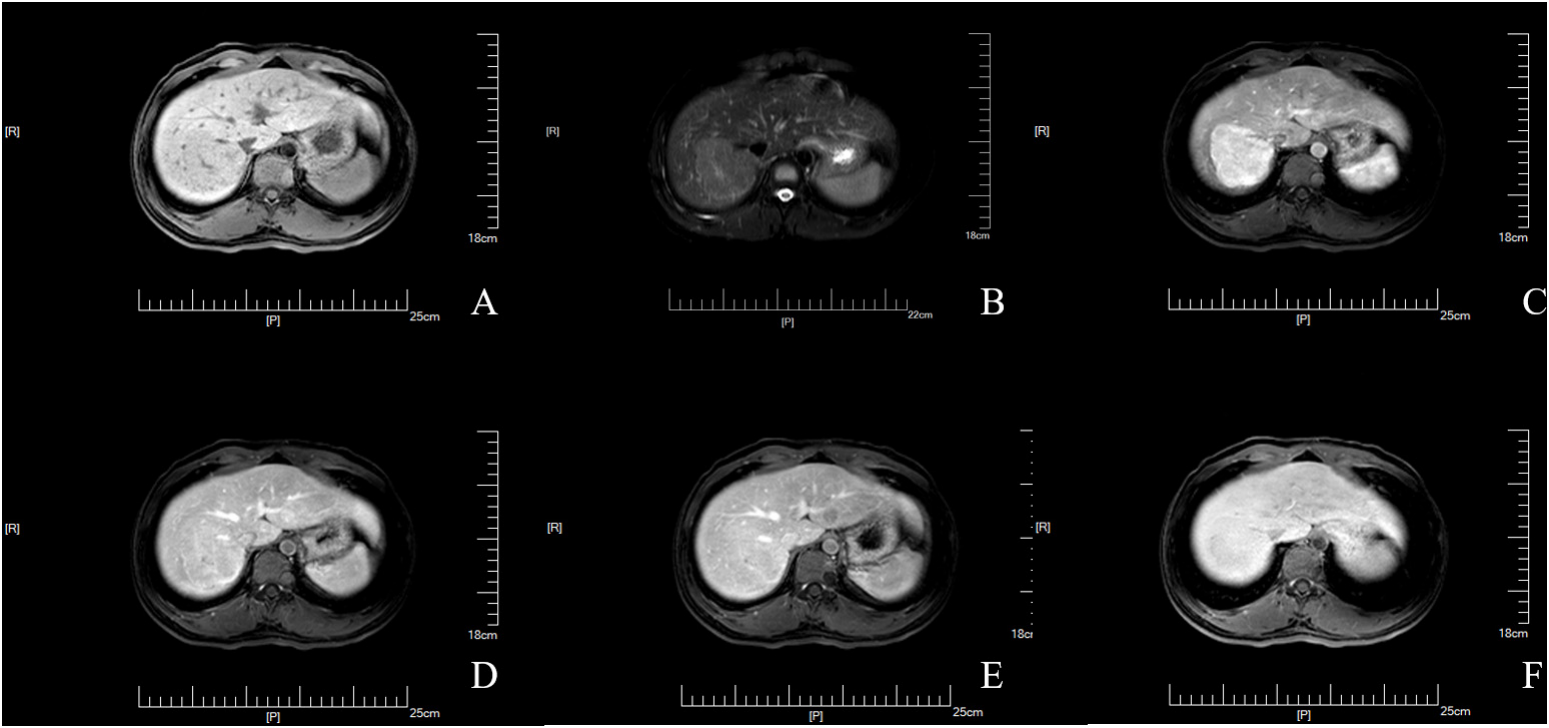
The lesion demonstrates typical imaging characteristics of FNH on Gd-EOB-DTPA-MRI. (A) T1-weighted image. (B) T2-weighted image. (C) Arterial phase. (D) Portal venous phase. (E) Transitional phase. (F) Hepatobiliary phase.
